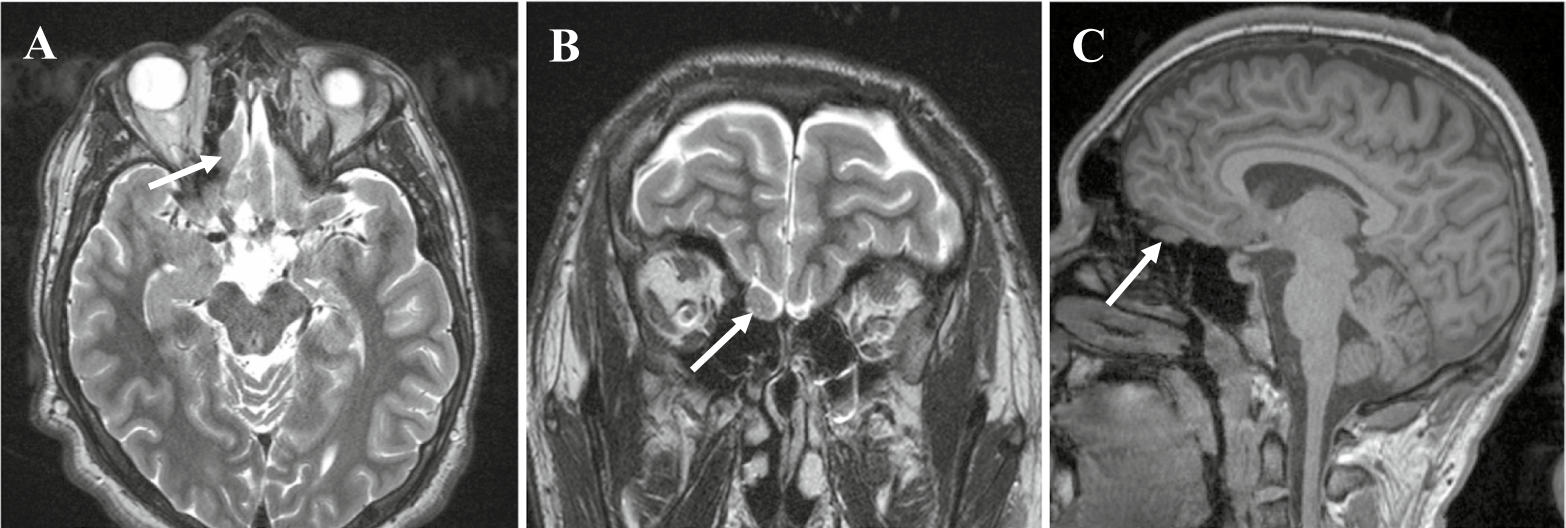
Axial (A) and coronal (B) preoperative T2-weighted and sagittal preoperative T1-weighted non-contrast (C) magnetic resonance images (MRI) demonstrating 0.8 × 1.0 cm ovoid mass in right olfactory tract (white arrows)
